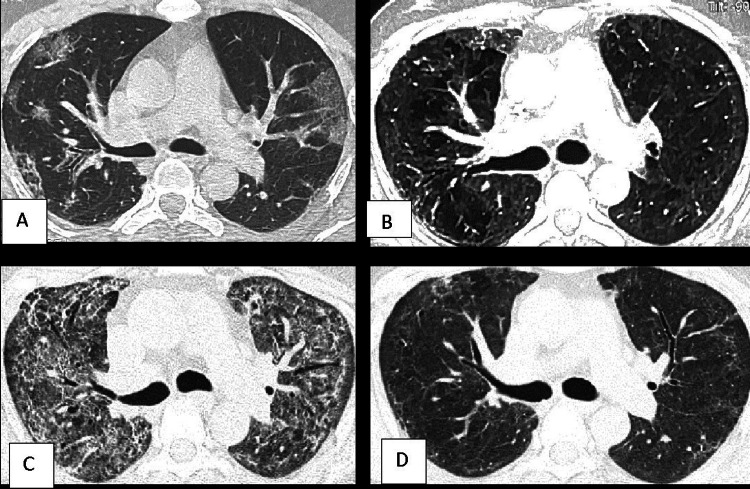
A 40-year-old male with waxing-waning lung CT opacities CT at initial diagnosis of COVID-19 shows geographically shaped, mainly peripheral, ground-glass opacities (GGOs) in both lungs (A). One and a half months later, CT shows a decrease in the geographic lesion, but a mild diffuse haziness throughout the lung parenchyma and few small reticular opacities are evident (B). Two and a half months later, significantly increased ground glass densities, bronchial dilatation, and reticular opacities are seen (C). And four and a half months later, there is a significant resolution of the density of the opacities, however, subtle diffuse haziness persists; note the walls of the earlier dilated bronchi are again smooth without the ‘wavy’ contour (D).
